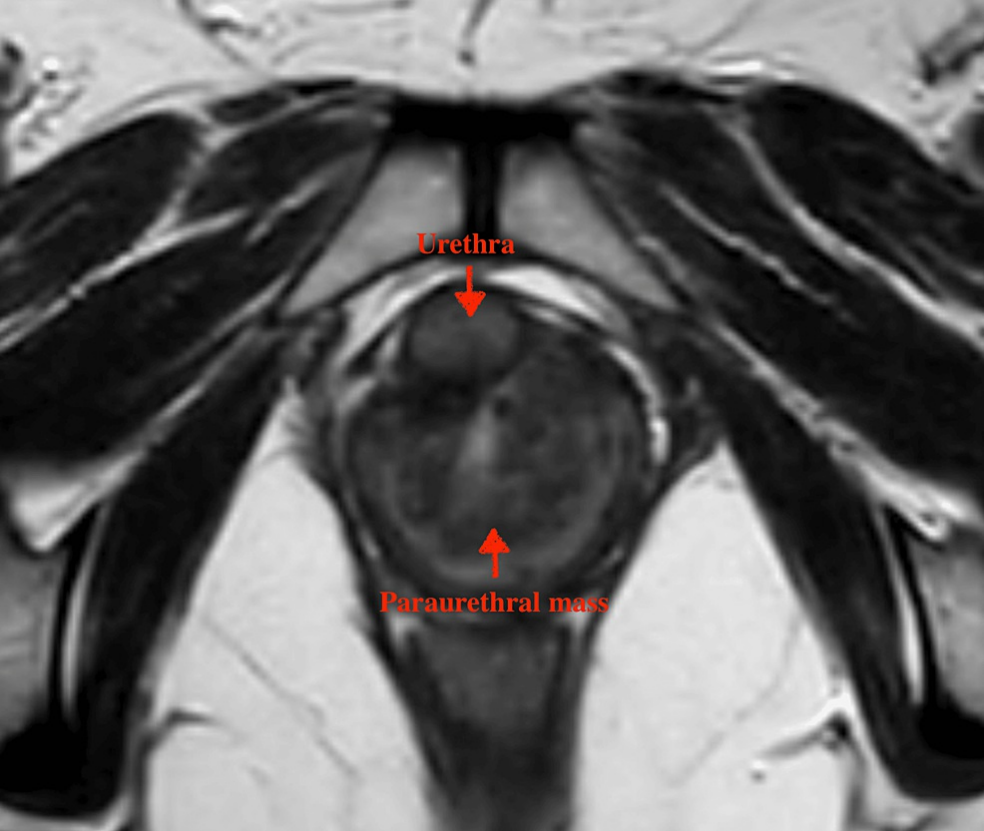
MRI showing the solid paraurethral mass in close relation to the urethra
